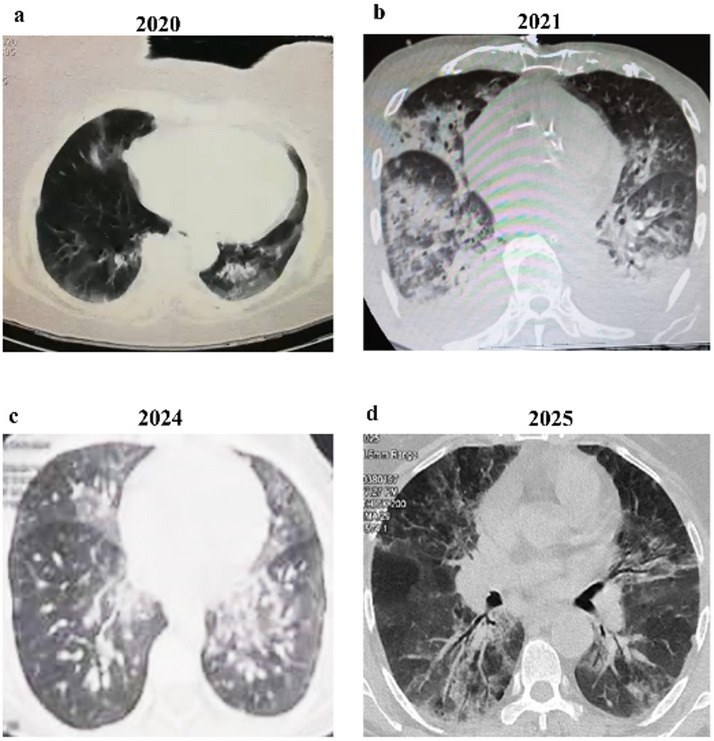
CT image showing GGO and consolidation (a), diffused bilateral GGO and consolidation (b), pulmonary lesions with a crazy paving pattern (c), tree-in-bud and bronchial wall thickening (d).
